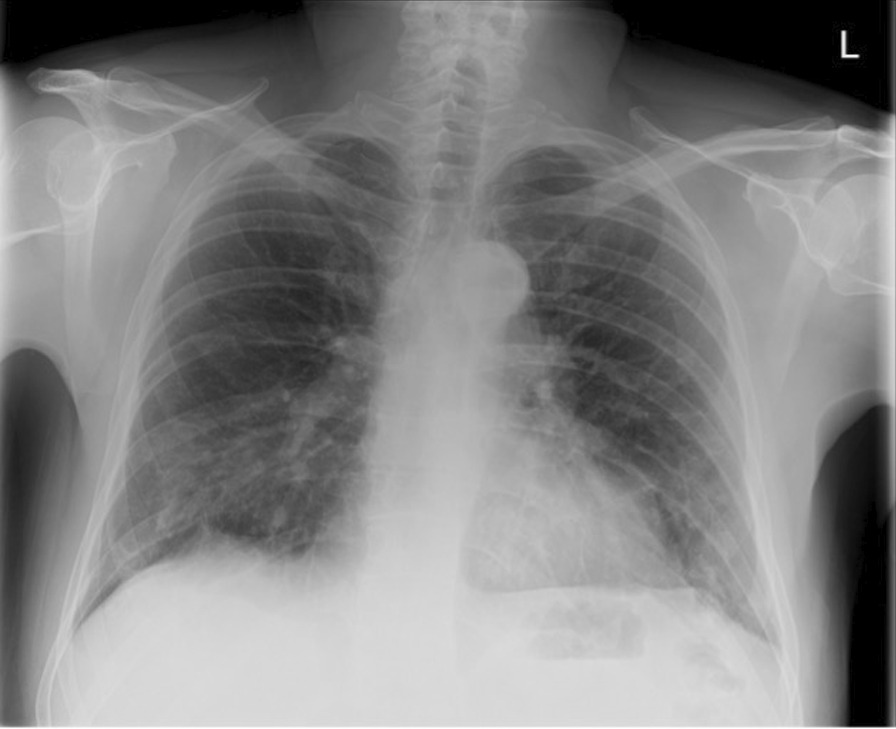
Chest X-ray on outpatient follow-up 1 month after discharge
